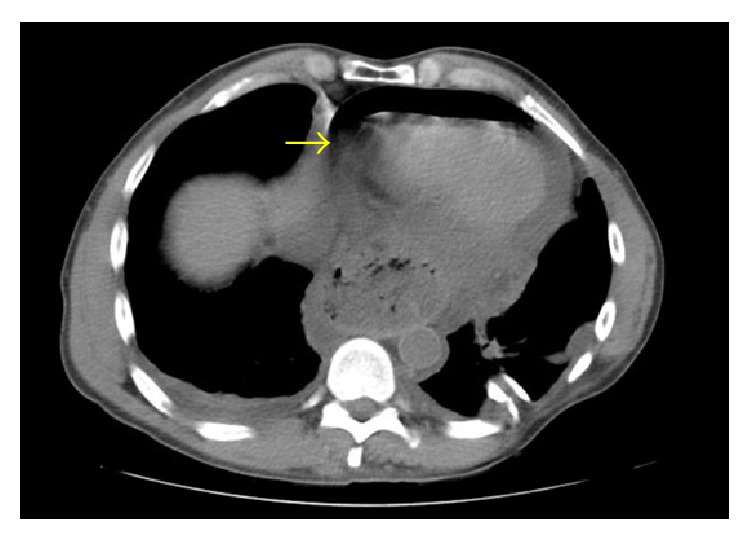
Chest computed tomography. There is an air fluid level in the pericardial space.
